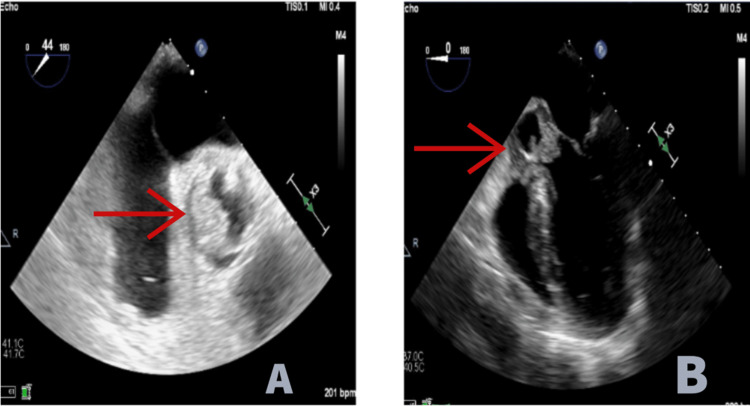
Vegetation of the aortic valve on TEE. A: Vegetations of the aortic valve at 44 degrees, short axis on TEE. B: Vegetation of the aortic valve at 0 degrees, mid-esophageal long axis on TEE. TEE = transesophageal echocardiogram
